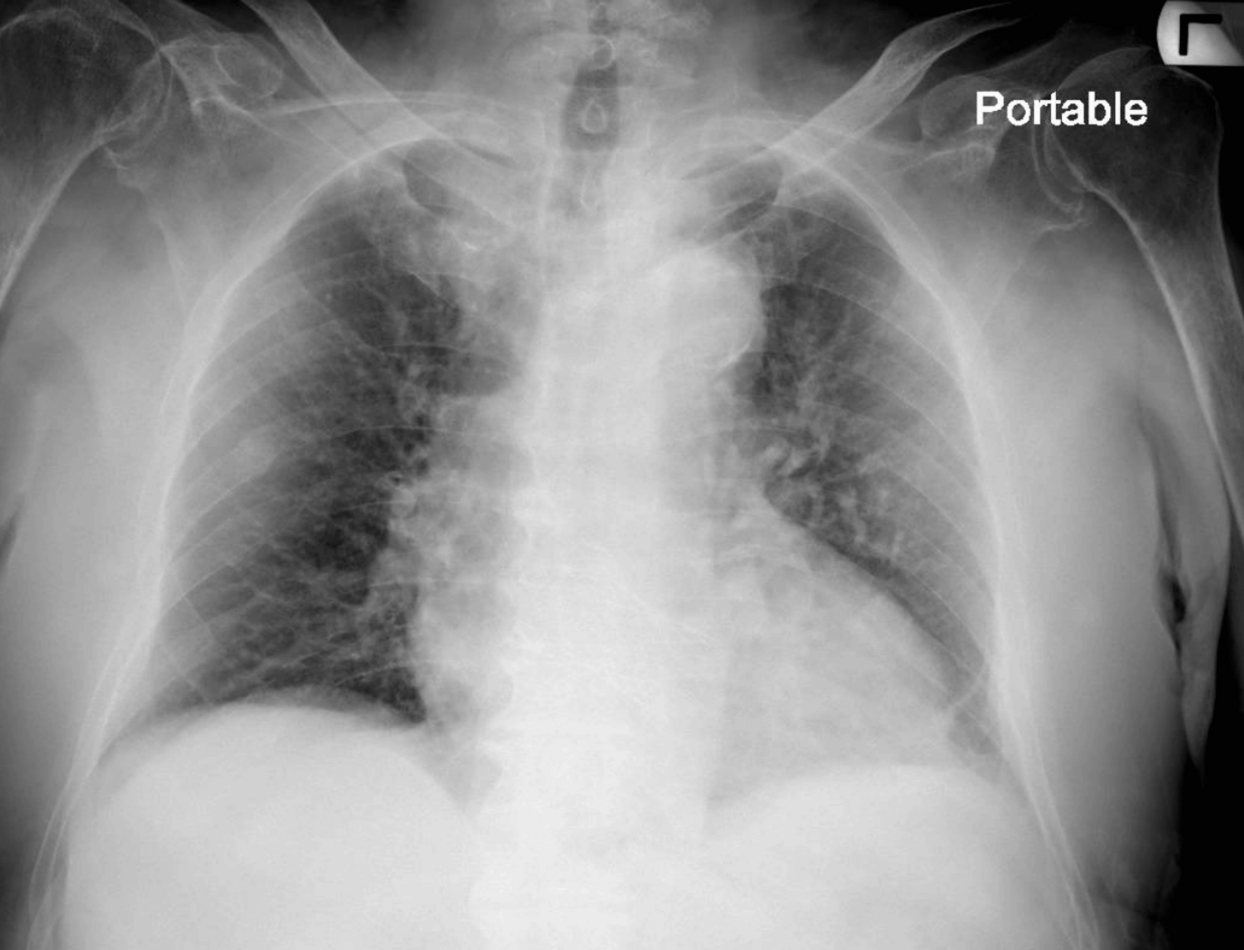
Portable one-view chest X-ray of the patient demonstrating a healed right-sided rib fracture likely from prior trauma, mild congestive heart failure, and no significant acute traumatic injury detected.
